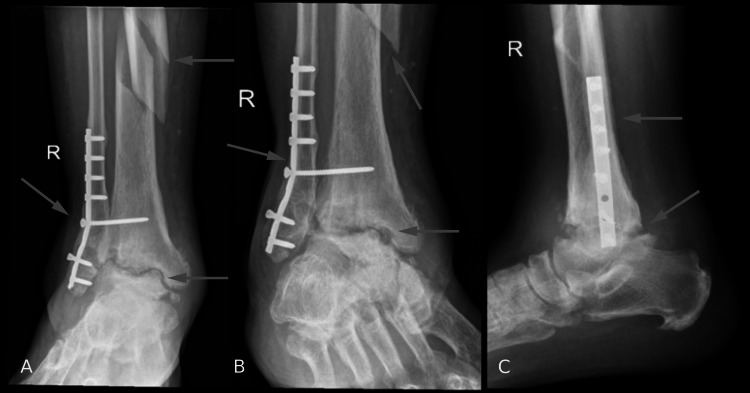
(A) Anteroposterior, (B) oblique, and (C) lateral X-rays of the ankle joint after the injury
